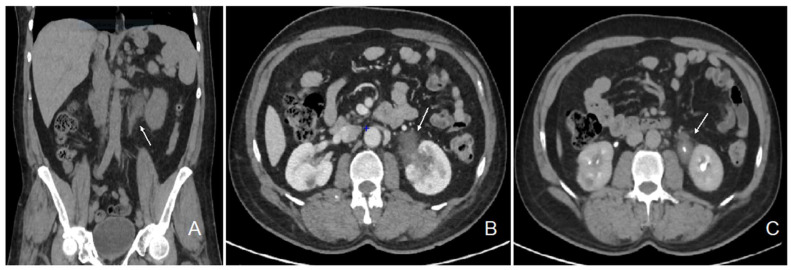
Abdominal CT scan images showing the pseudotumor of the left renal pelvis. Legend: coronal section, non-contrast phase (A); transversal section, nephrographic phase (B); transversal section, excretory phase (C); left ureteral tumor (white arrow).
